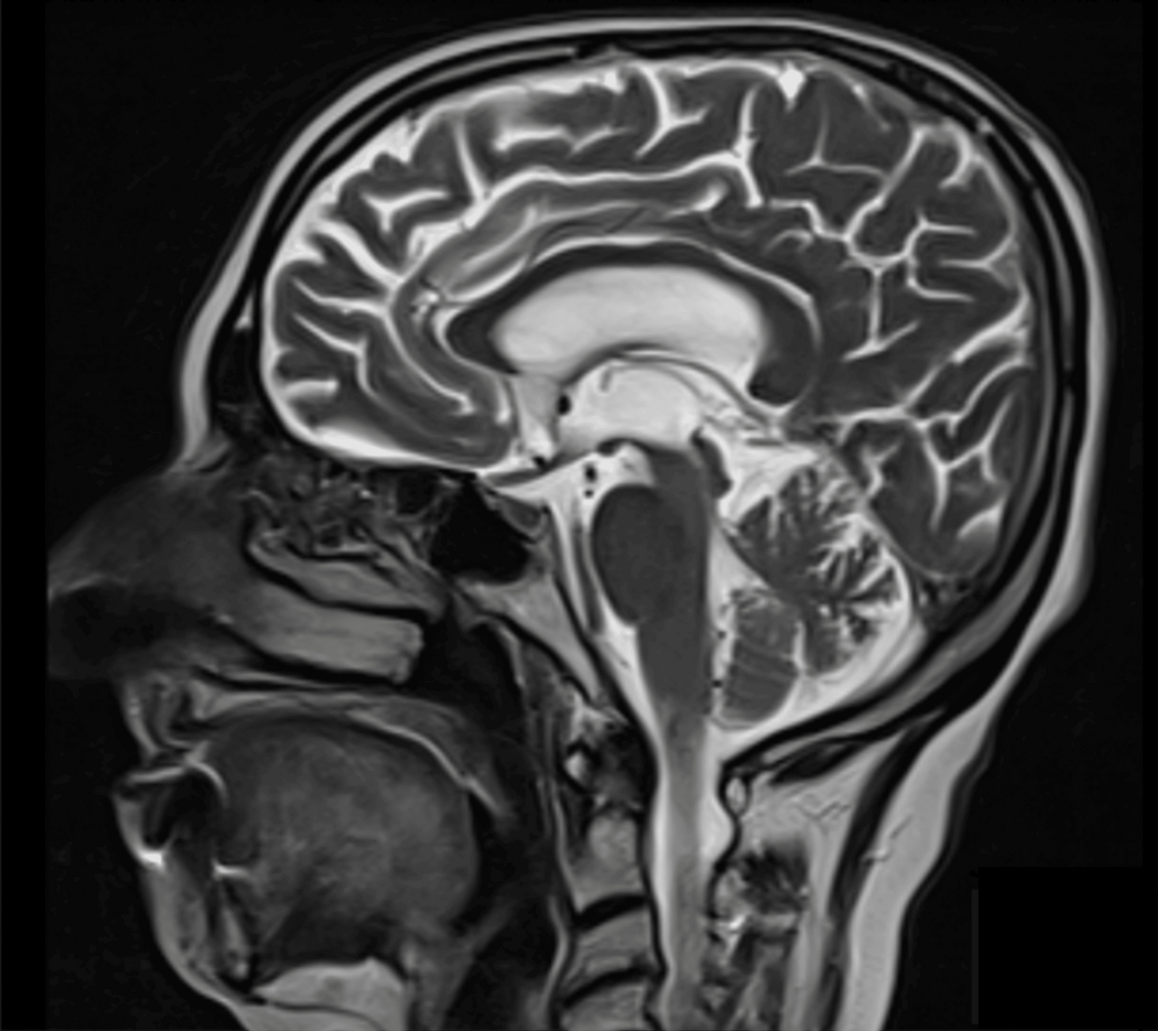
The sagittal T2-weighted MRI showing selective and pronounced atrophy of the midbrain, with mesencephalic predominance and preservation of the pons, characteristic of the “hummingbird sign” in PSP. The midbrain-pons ratio is 0.43 (which, when less than 0.52, demonstrates 100% specificity for PSP). The midbrain-pons area ratio is 0.14 (a value less than 0.16 is suggestive of PSP). PSP, progressive supranuclear palsy
